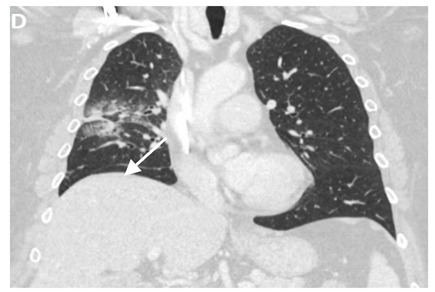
Same patient post-radiation therapy coronal CT chest (image D), 1.5 months later showing ground-glass opacity and patchy airspace consolidation in RML conforming to the area of radiation field denoted by an arrow.
